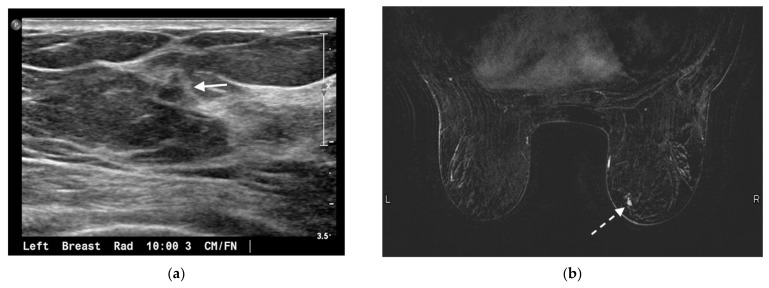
75-year-old woman presented for annual screening evaluation. Mammogram demonstrated a left breast asymmetry (not shown) which prompted further workup. (a) Left breast ultrasound in radial projection at the 10 o’clock axis 3 cm from the nipple demonstrates a suspicious 0.5 × 0.6 × 0.4 cm hypoechoic antiparallel mass with irregular margins and posterior acoustic shadowing (arrow), corresponding to the mammographic abnormality. Ultrasound guided biopsy yielded invasive ductal carcinoma, mucinous type. (b) MRI was performed to evaluate the extent of disease; axial contrast-enhanced T1-weighted fat-suppressed image demonstrates a separate 0.7 × 0.5 cm enhancing irregular mass in the lower inner quadrant of the contralateral right breast (dashed arrow). This was biopsied under MRI guidance and yielded invasive ductal carcinoma, mucinous type. The known left breast cancer is not shown.
